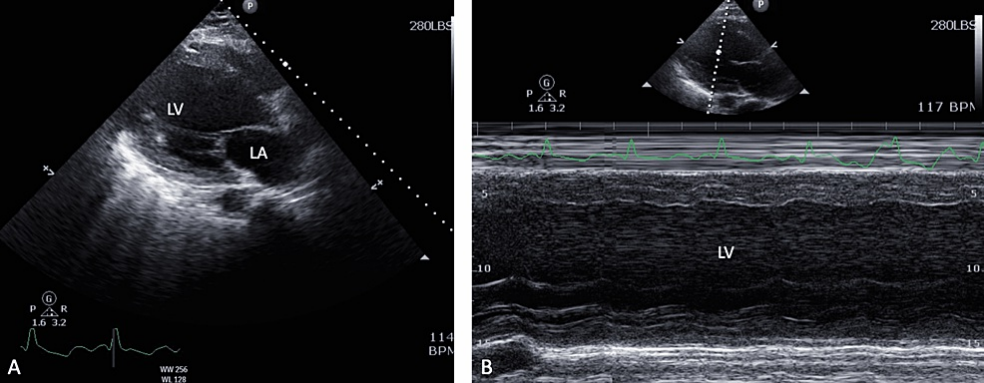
(A) Parasternal axis view of TTE showing severely dilated LA and LV. (B) M-mode showing severely dilated LV with poor LV function. TTE, transthoracic echocardiogram; LA, left ventricle; LV, left ventricle
